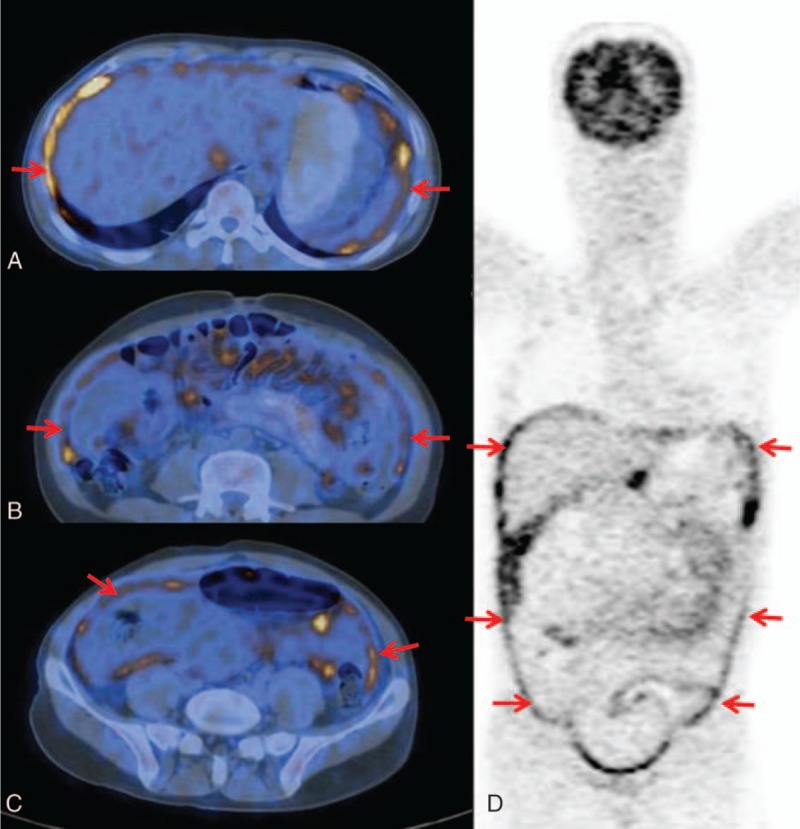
A 37-year-old man with tuberculous peritonitis. Diffuse involvement and uniform distribution in the parietal peritoneum: string-of-beads ^18^F-FDG uptake (red arrow) and uniform distribution in the bilateral subdiaphragmatic regions (A), the bilateral paracolic gutter regions (B), and the pelvic region (C) on combined PET/CT axial imaging; string-of-beads ^18^F-FDG uptake (red arrow) in all 5 regions of the parietal peritoneum on whole-body PET coronal-sectional imaging (D). CT = computed tomography, ^18^F-FDG = F18-fluorodeoxyglucose, PET = positron emission tomography.
